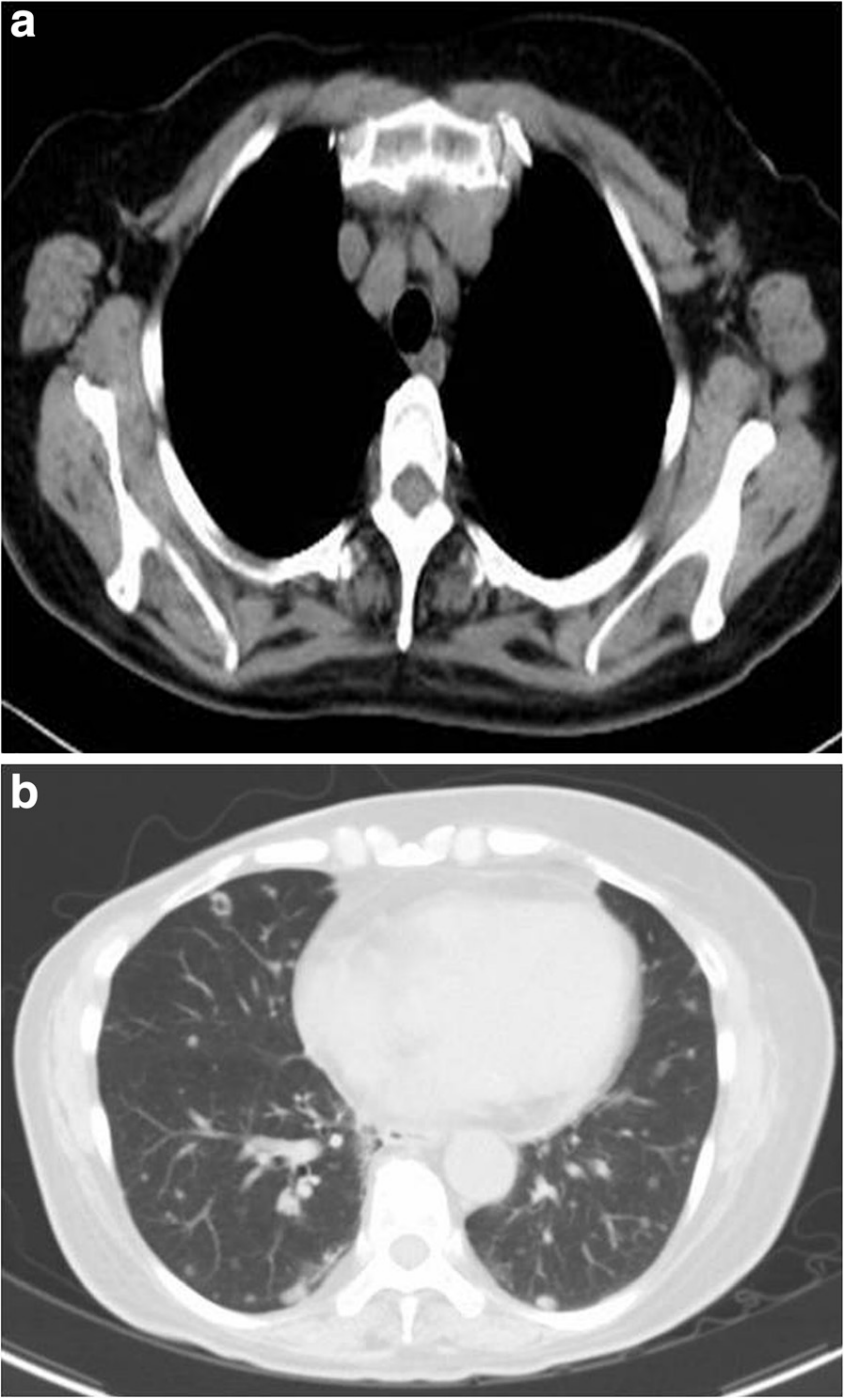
a and b (CT from April 2012). Three months after bevacizumab and weekly PTX were begun, the left axillary lymph node reduced in size to 1.4 cm and the lung metastases also became smaller.
